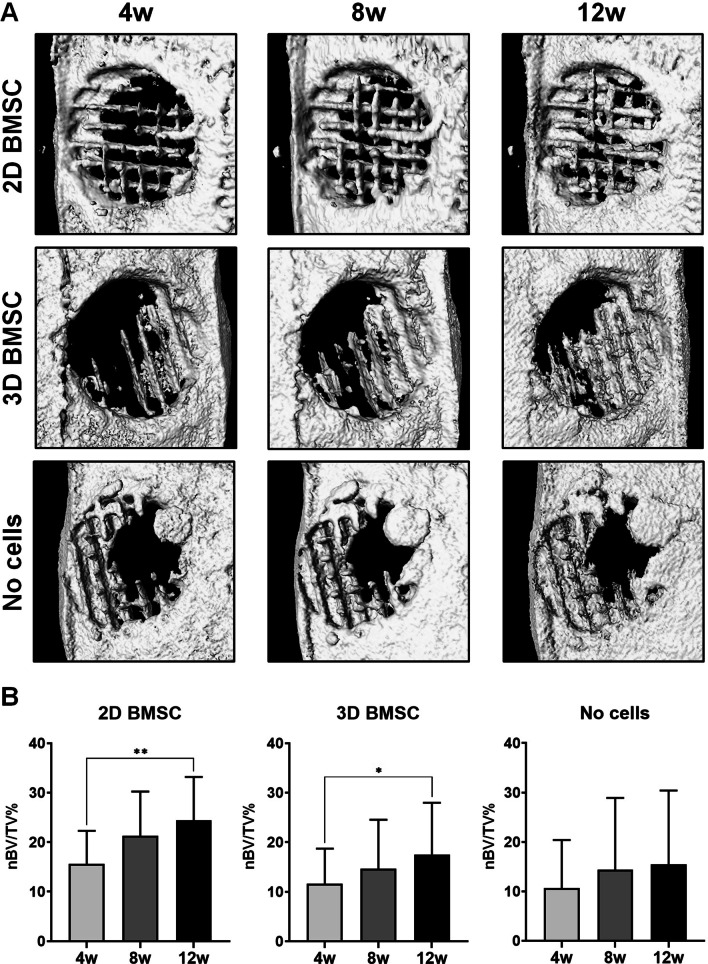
In vivo CT scanning. Representative reconstructed CT scans showing progression and distribution of bone regeneration (a) and corresponding quantification (b) in constructs with 2D BMSC, 3D BMSC and no cells from 4 to 12 weeks (w); data represent means ± SD; *p* < 0.01; p* < 0.05
